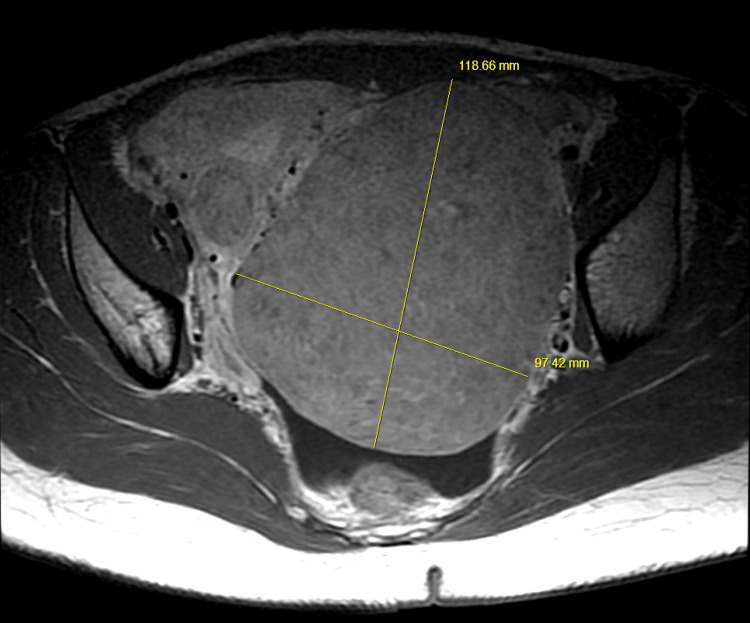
Axial pelvic MRI showing a large uterine fibroid measuring approximately 120 x 100 mm. Structures (sciatic nerve) in between the fibroid and piriformis muscle on the left side appear compressed compared to the right side.
